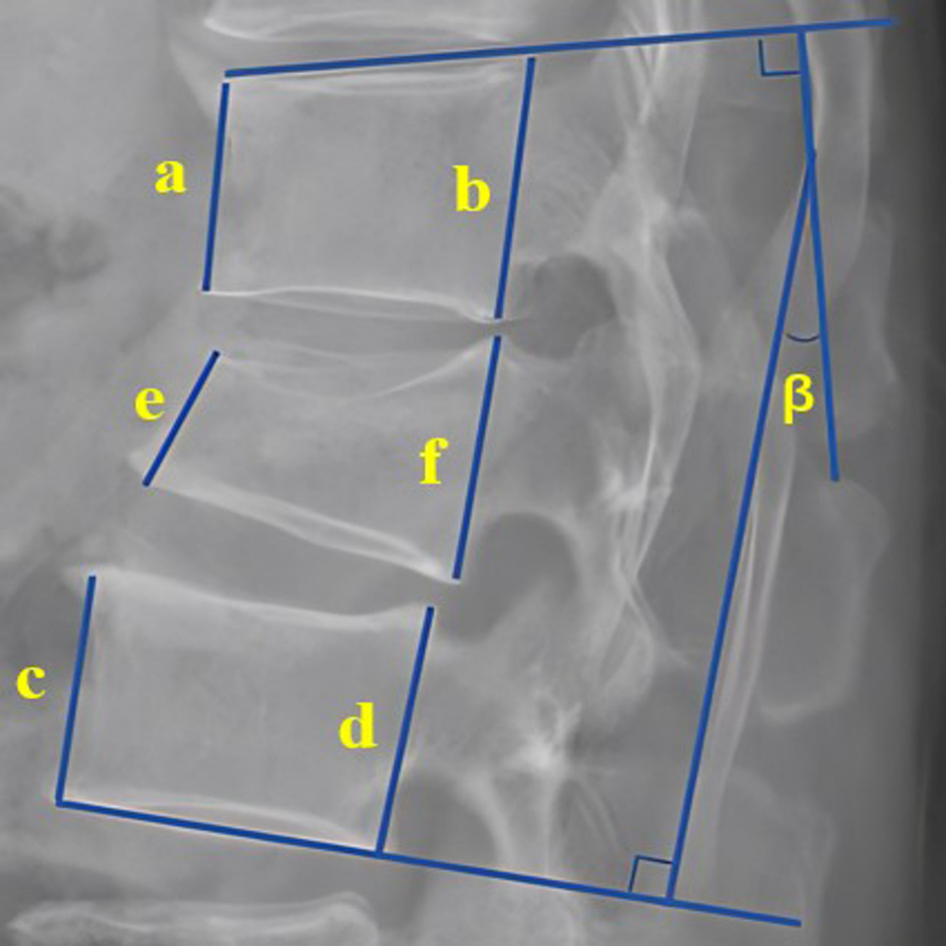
Measurement method for VHL and KA. (1) The KA (*β*) was measured as the angle between the superior endplate of the vertebra above and the inferior endplate of the vertebra below on lateral radiography. (2) (a) Anterior height of the cranial vertebrae, (b) posterior height of the cranial vertebrae, (c) anterior height of the caudal vertebrae, (d) posterior height of the caudal vertebrae, (e) anterior height of the fractured vertebrae, (f) posterior height of the fractured vertebrae. VHL =
